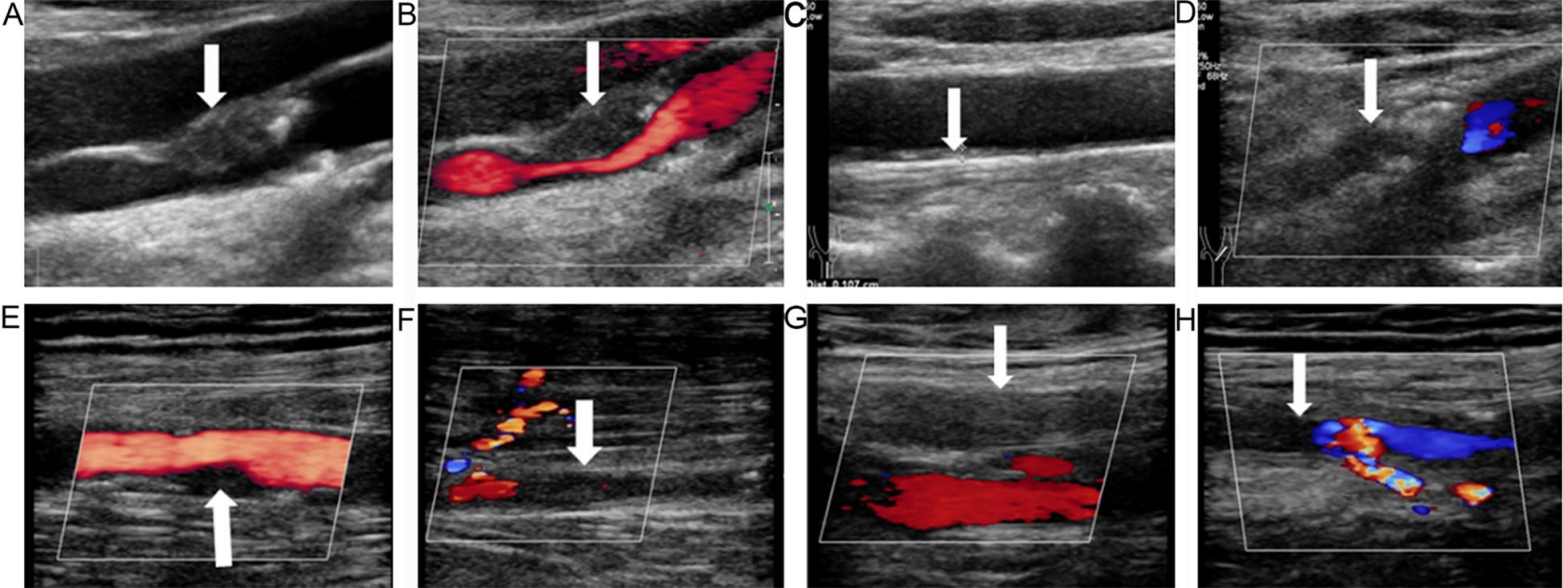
Ultrasonography of the carotid and lower limb arteries of a 74-year-old male patient with diabetic foot, peripheral arterial disease, and plaques in both internal carotid arteries. A, High-resolution gray-scale sonography revealed a plaque (arrow) in the front wall of the right internal carotid artery, and C, an increase in the intima-media thickness of the left common carotid artery (arrow; the measured value was 1.07 cm). B, Color Doppler ultrasound showed severe stenosis of the lumen of the right internal carotid artery (arrow; the diameter was reduced by 70–99%), D, indicated that the left internal carotid artery was occluded (arrow), E, moderate stenosis of the right superficial femoral artery (arrow; the diameter was reduced by 50–99%), F, segmental occlusion in the anterior tibial artery (arrow) along with a collateral artery, G, occlusion of the left superficial femoral artery (arrow), and H, occlusion of the left superficial femoral artery (arrow) accompanied by a collateral artery. The overall ultrasonic score was 10 points for the right lower limb and 9 points for the left lower limb.
